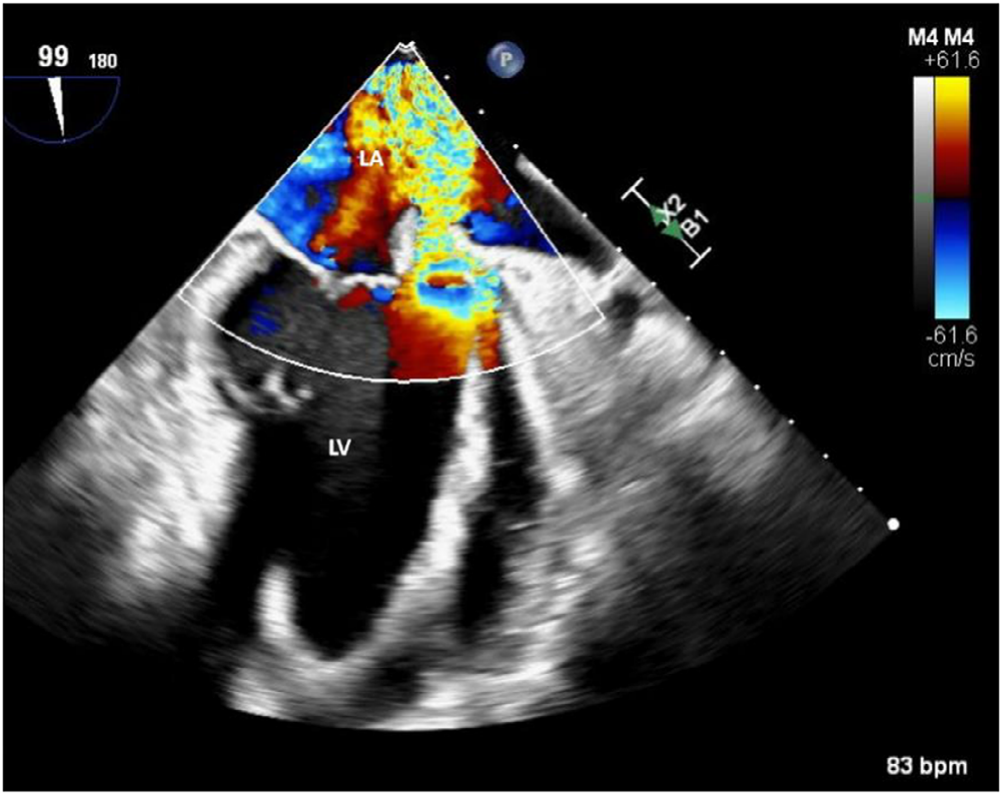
Transesophageal echocardiogram with color Doppler demonstrating severe mitral regurgitation secondary to valvular destruction from pneumococcal endocarditis. LA, left atrium; LV, left ventricle.
